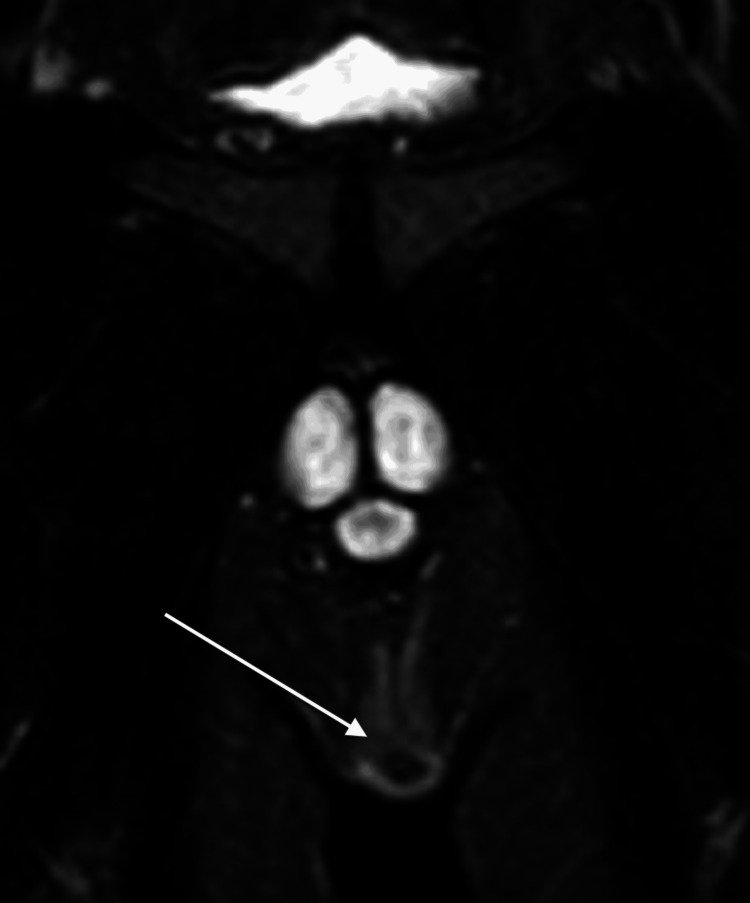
Pelvic MRI showing a small superficial collection (arrow) within the subcutaneous fat of the left perineum
